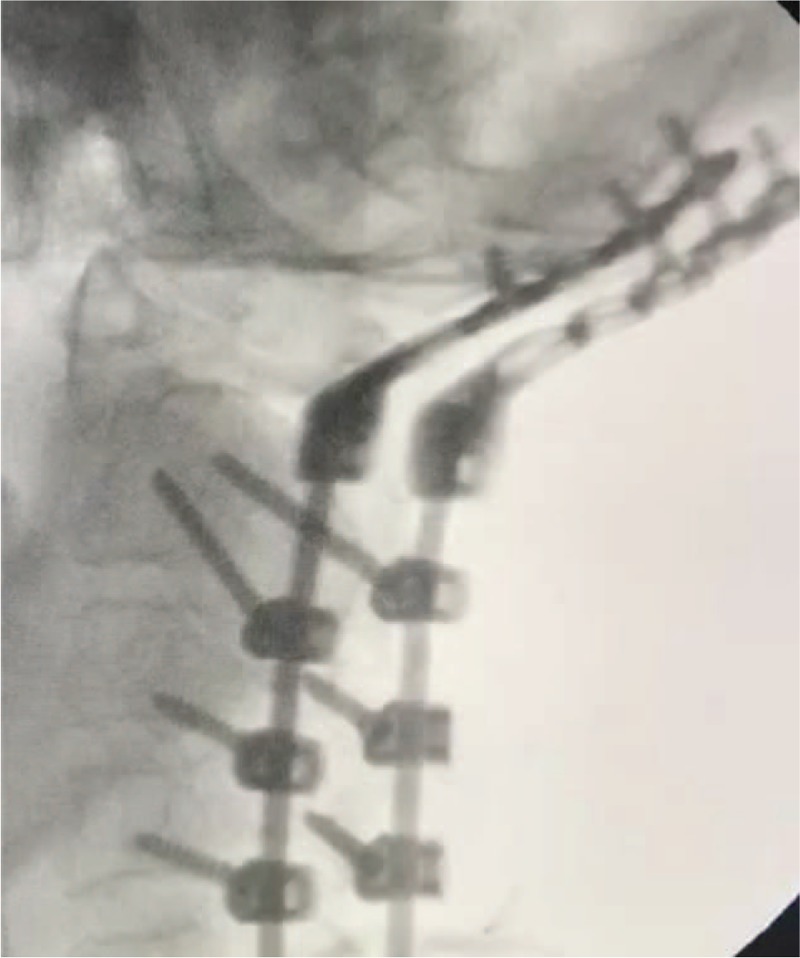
Intraoperative X-ray showing that the screws are in the correct position without displacement.
